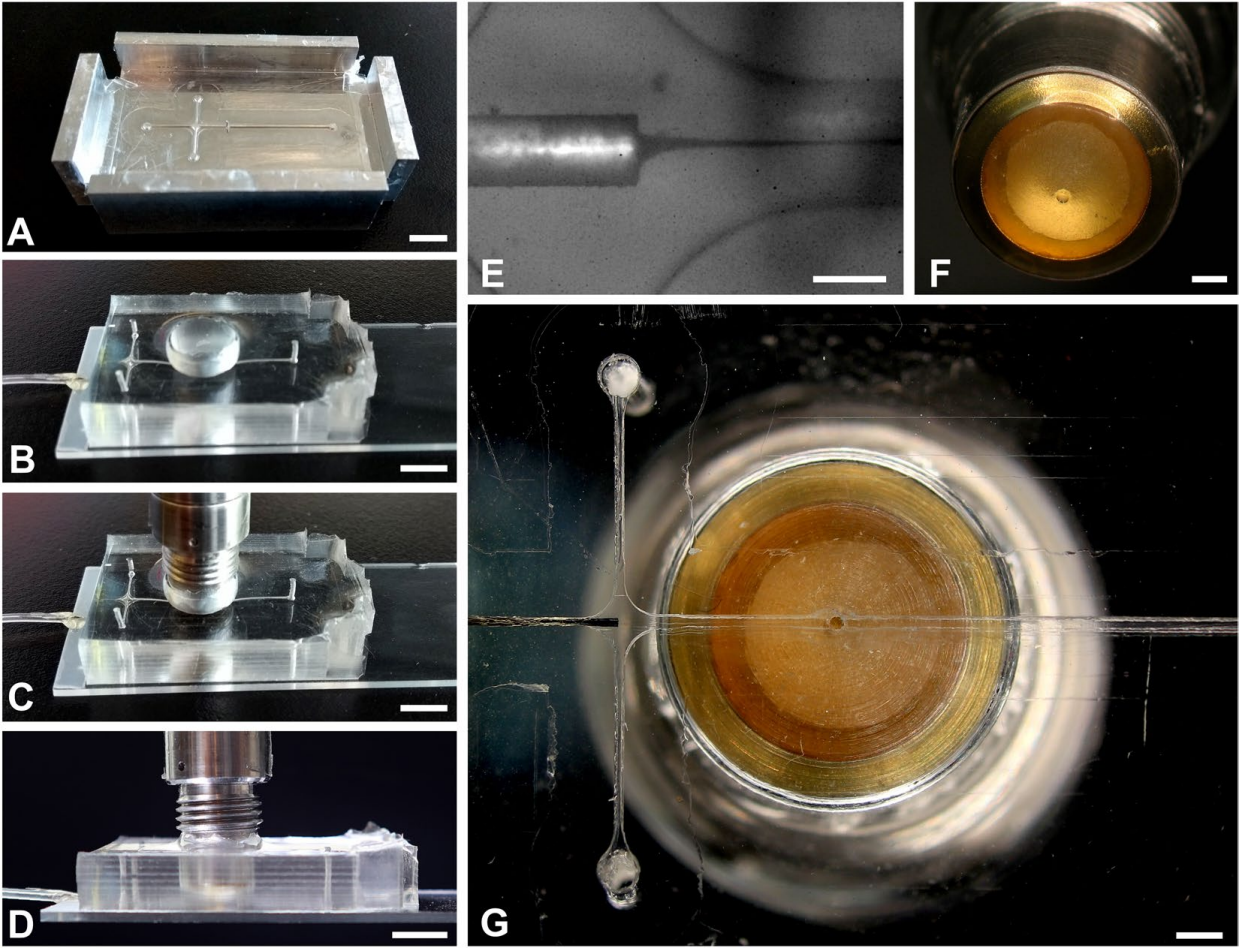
The PDMS flow focusing device. (A) The mold used to cast the PDMS. (B–D) The PDMS device bonded to the glass slide with the ultrasound transducer aligned to the focusing stream. (E) Optical image showing the flow focusing capability using black ink. (F) The ultrasound transducer, the aperture tip is 300 μm in diameter. (G) A bottom view of the PDMS device with the transducer aligned to the center of the flow channel. Scale bars: (A–D) 5 mm, (E) 200 μm, (F,G) 1 mm.
